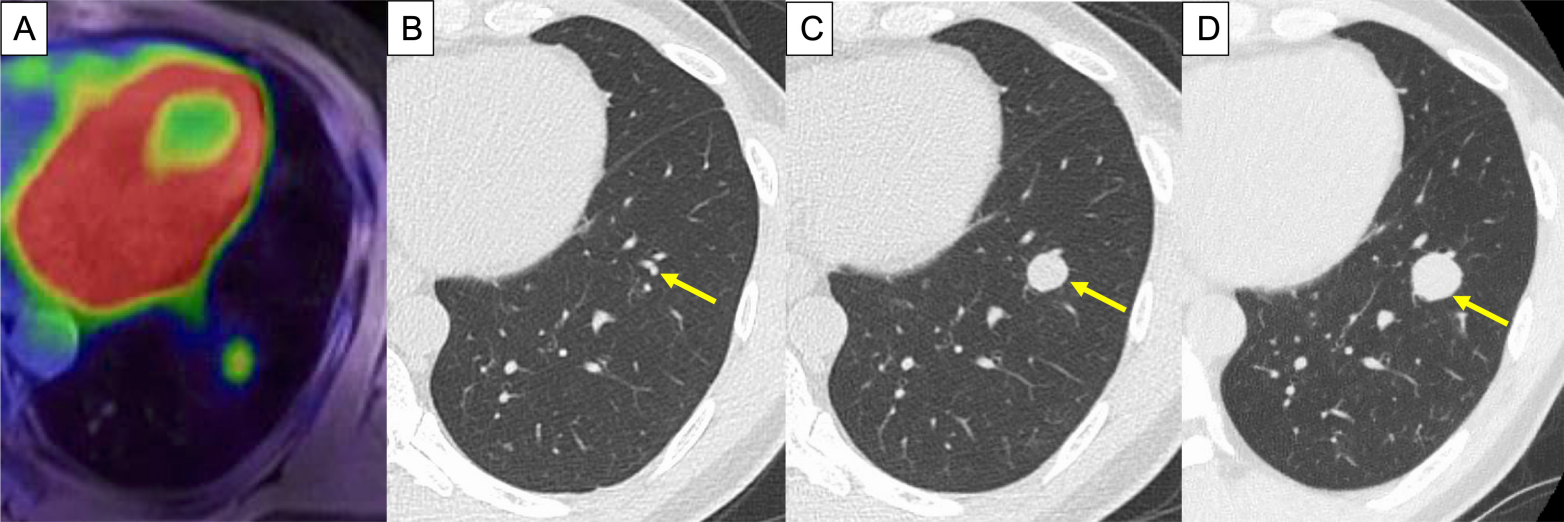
(A) Positron emission tomography‐magnetic resonance imaging (PET‐MRI) shows a solid tumour with fluorodeoxyglucose uptake in the left lower lung. (B) Retrospectively reviewed computed tomography (CT) image reveals a 3‐mm nodule 1 year prior to detection. (C) The patient was referred to our hospital and CT was performed. The CT image reveals a well‐defined 13‐mm nodule in the left S9 segment. (D) A follow‐up chest CT was performed 1 month later. The CT image demonstrates rapid growth to 18 mm, with homogeneous contrast enhancement.
